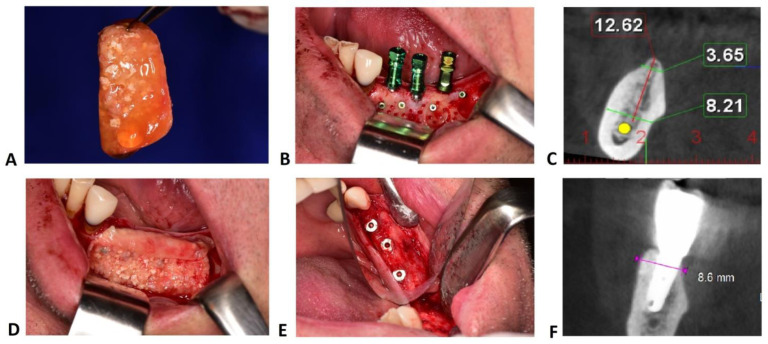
Sticky bone technique. (A) Sticky bone, a solid aggregate composed by particulate xenograft mixed with autograft, immersed in fibrin gel concentrated with growth factors, moulded in the desired shape. (B) Recipient bed prepared with retentive osteosynthesis screws and implants inserted in the correct prosthetic position. (C) Preoperative measurements on CBCT sections of the area of interest. (D) Bone graft material hanged on the osteosynthesis screws. (E) Regeneration area uncovering after 6 months. (F) Post-operative CBCT of the augmented ridge with sticky bone.
